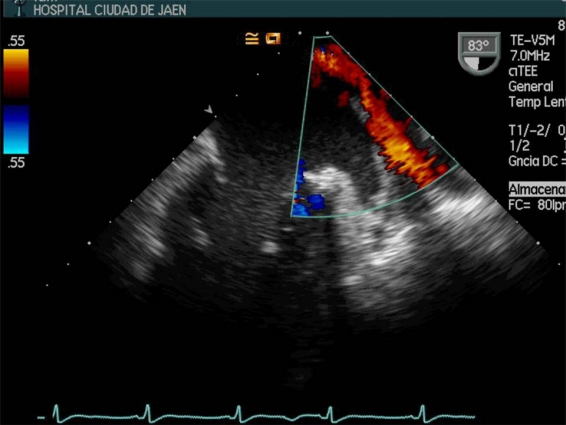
Transesophageal image projection which shows the pulmonary veins flow. Quantifying the systolic filling fraction, left atrial pressure can be calculated. (LA: Left atria. LV: Left ventricle. LAp: left atrial appendage).
